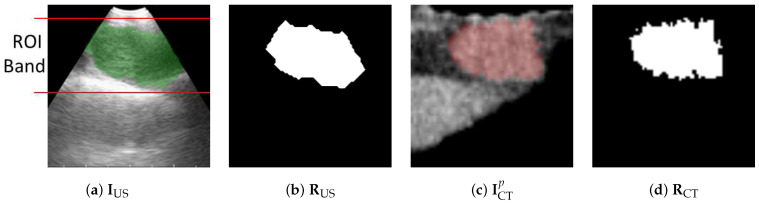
Limiting region of calculations for (5) in cost $C_{N}$. (a) Trapezoidal EBUS region delineated by the lines encompassing the EBUS ROI in $I_{\mathrm{US}}$ with (b) showing segmented ROI $R_{\mathrm{US}}$. (c,d) Corresponding virtual EBUS view $I_{\mathrm{CT}}^{p}$ and predefined ROI $R_{\mathrm{CT}}$.
